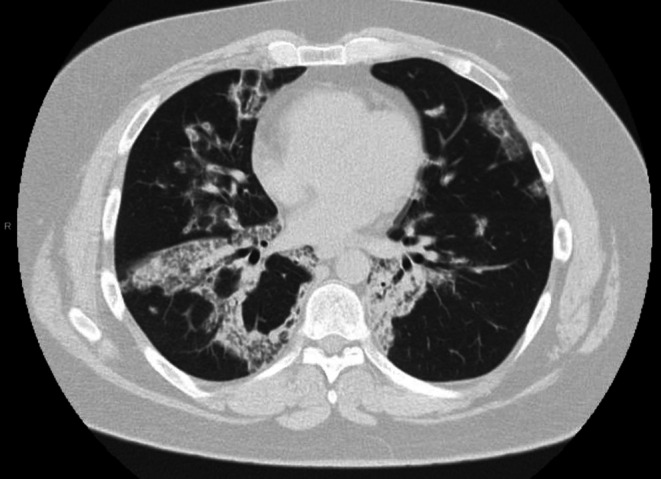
CT scan showing decreasing burden of pulmonary alveolar proteinosis with significantly improved clearance of the bilateral lower lobes.
